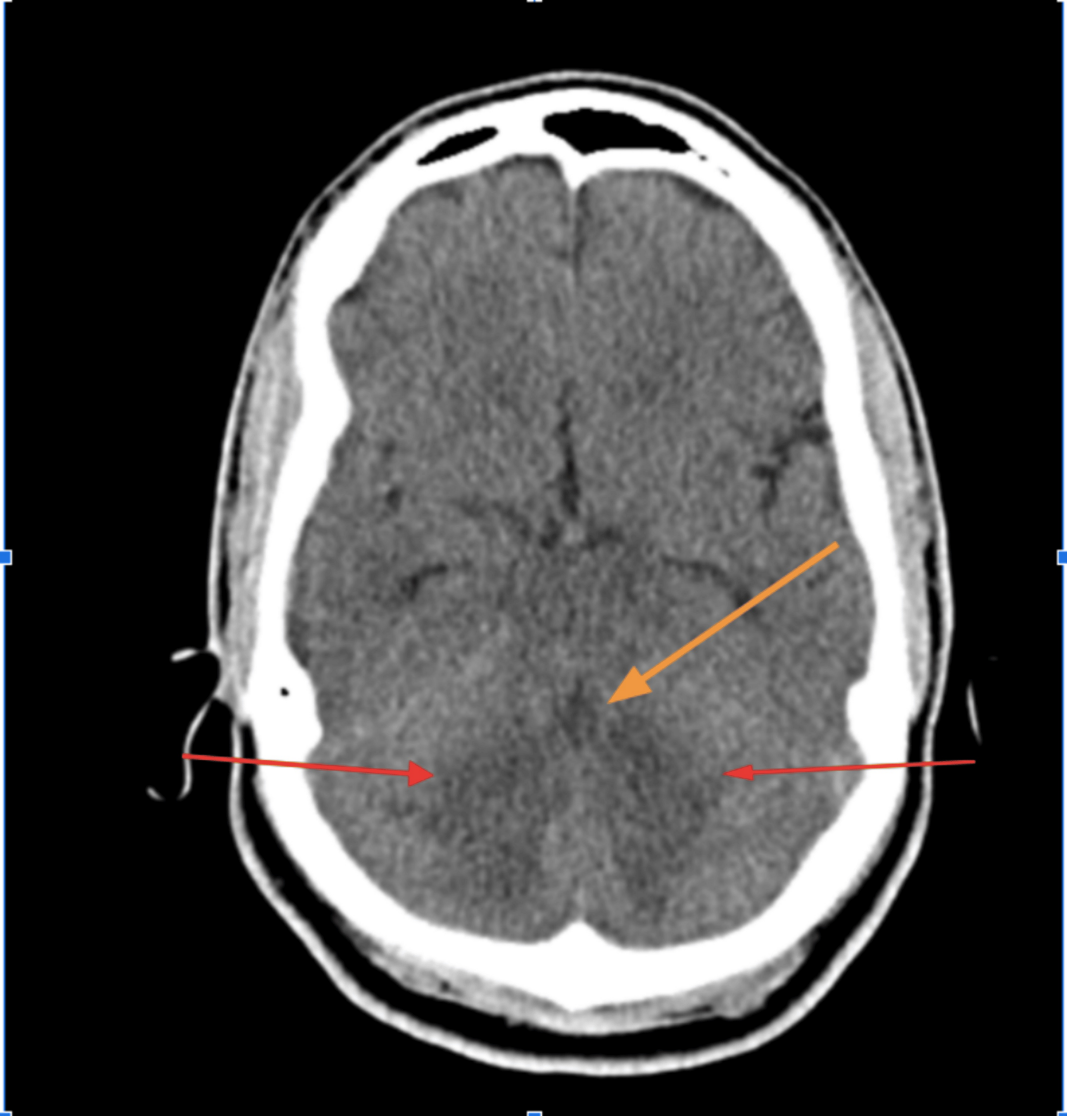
CT of the brain without contrast (axial view) The image shows a large hypodense area involving the cerebellum representing cerebellar edema (red arrows) and mild localized mass effect with partial effacement of the fourth ventricle shifted slightly to the right of midline (orange arrow). CT: computed tomography
